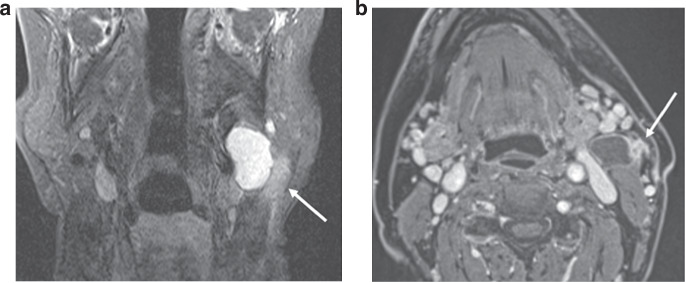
Iatrogenic changes post core biopsy—example 1. HPV-positive OPSCC, 6 days following core biopsy of a cystic left Level II node. a Coronal STIR MRI; b Axial T1FS+c MRI, showing minor localised perinodal stranding and enhancement at the lateral margin of the node (arrows). The position corresponds to the core biopsy needle path.
